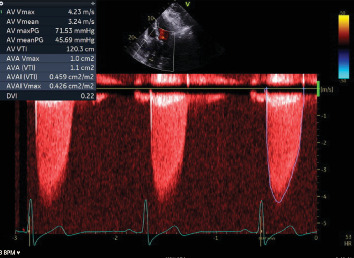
Continuous wave Doppler tracing across aortic valve demonstrating severe aortic stenosis.
